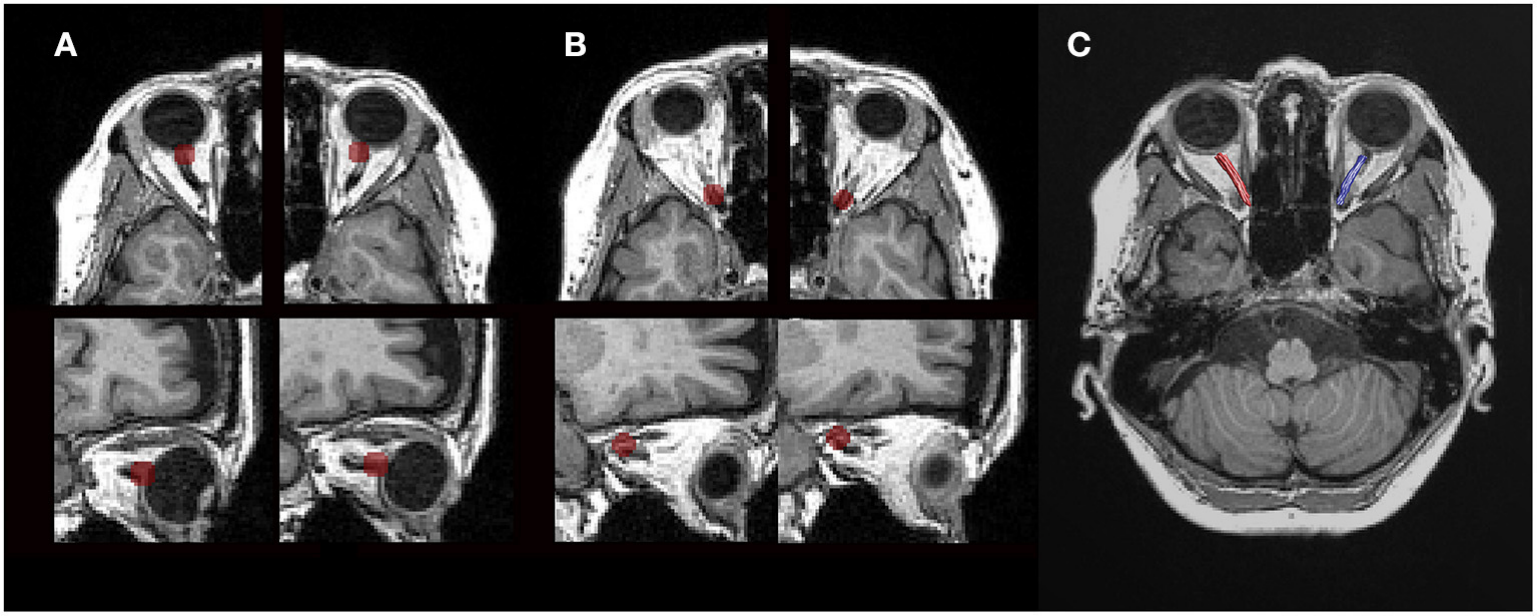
Tractography using diffusion magnetic resonance imaging. (A) Axial and sagittal views (top to bottom) of the regions of interest (ROIs). A 4-mm-radius spherical ROI just posterior to the globe was centered on the emerging optic nerve in each eye. (B) Another 4-mm-radius spherical ROI was within the orbital apex, and its center was placed in the center of the annulus of Zinn. (C) Cleaned tractography-generated optic nerve pathways. Visualization of the ROIs and tractography was generated with the MATLAB Brain Anatomy software package (https://github.com/francopestilli/mba).
